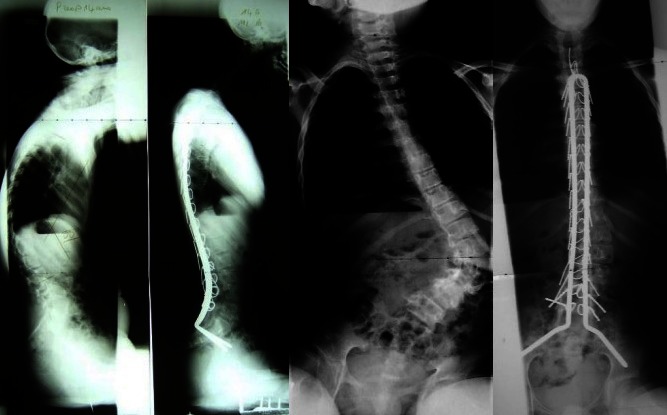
Pre and postoperative X-Rays for a Cerebral Palsy patient surgically treated by the Unit Rod method
